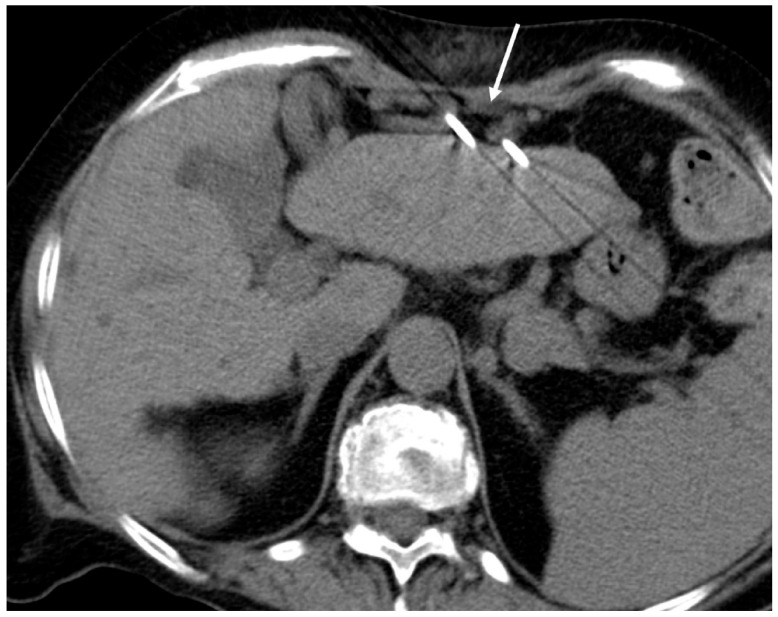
Left: Axial CT scan shows the accidental transgression/close proximity of the ablation needle through the bowel loops (arrow).
